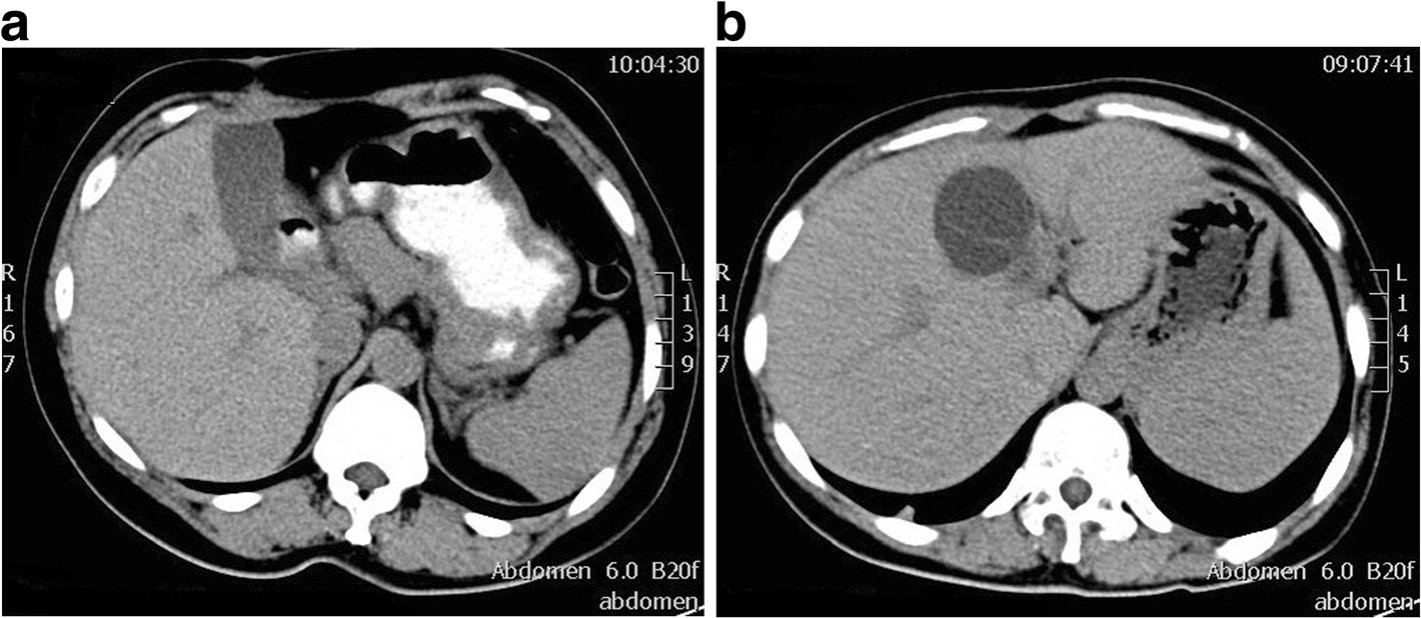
Imaging of epigastric computed tomography 1 year after the open operation: a and b show a normal hepatic duct and a similar-sized hepatic cyst compared with preoperatively
